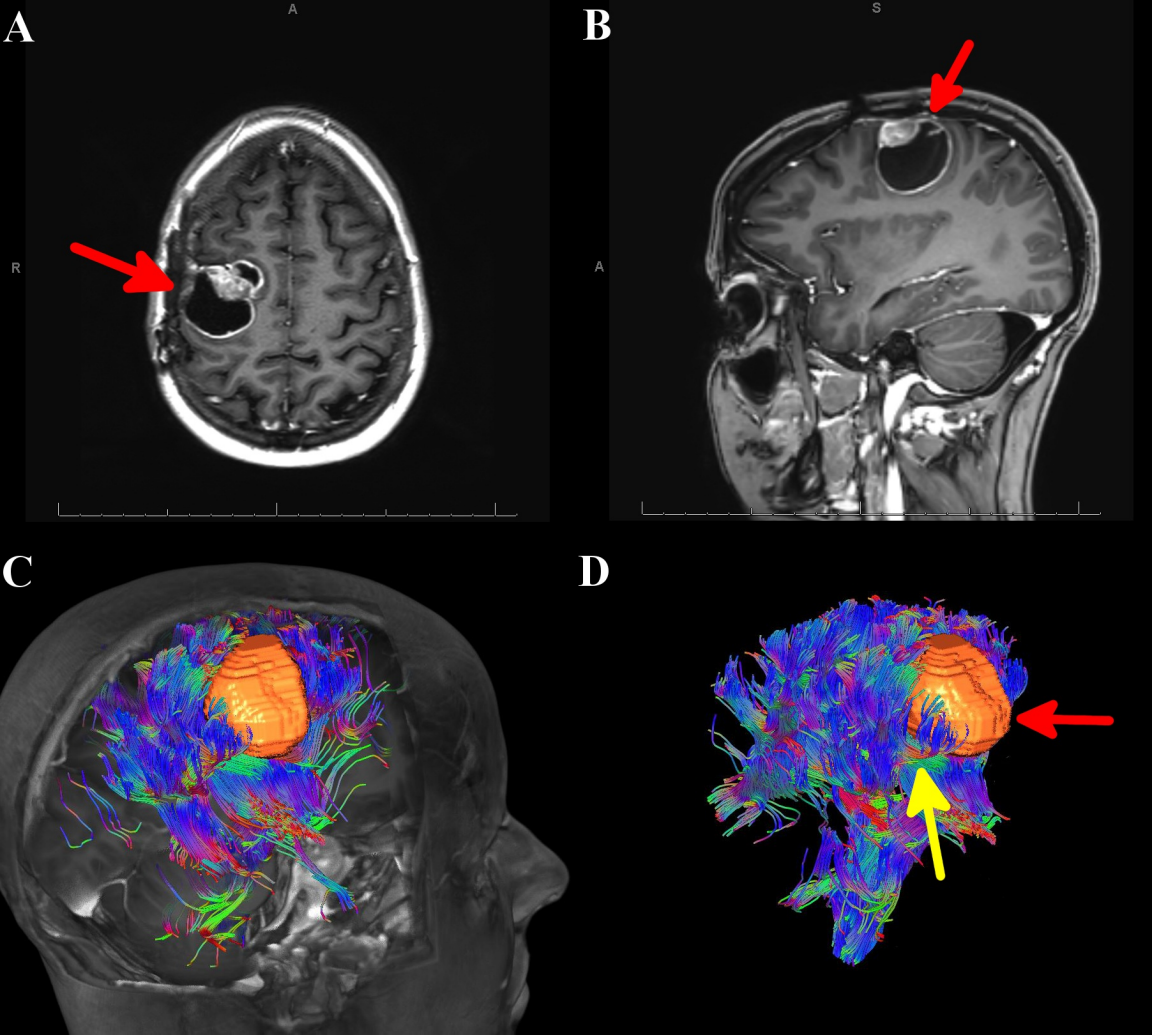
MRI of the brain and automated whole brain tractography (AWBT) for Case 1 A) Axial MRI of the brain with contrast showing a right-sided mass, denoted by a red arrow. B) Sagittal MRI of the brain with contrast showing a right-sided mass, denoted by a red arrow. C) AWBT image showing the tumor to be sitting within the corticospinal tract. D) AWBT image showing the tumor (denoted by red arrow), sitting within the corticospinal tract (denoted by yellow arrow). AWBT: automated whole brain tractography; MRI: magnetic resonance imaging
